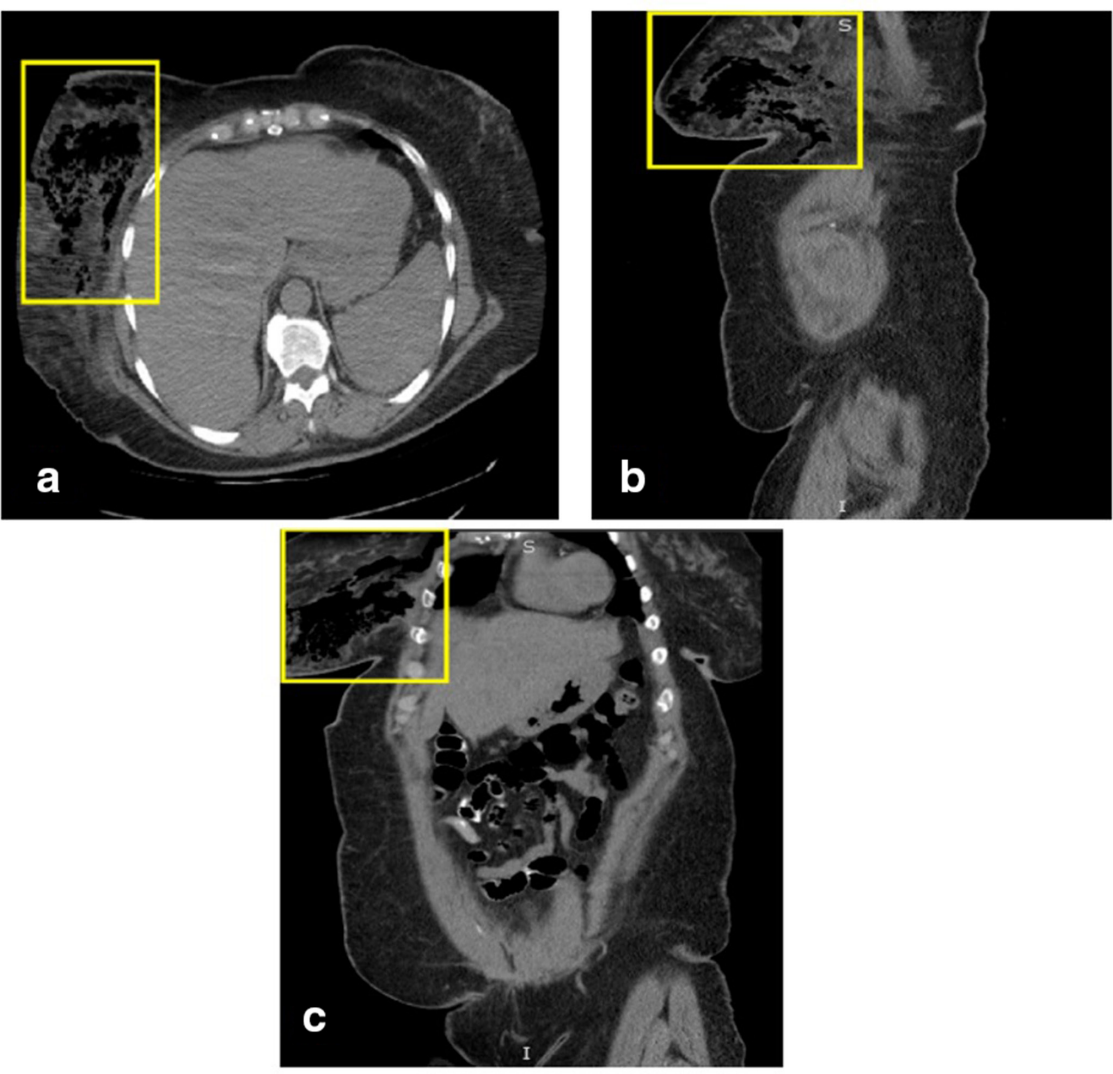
CT imaging displays the reported inflammatory changes and subcutaneous emphysema of the right breast. A displays an axial view with the yellow box demonstrating significant inflammatory changes and subcutaneous emphysema in the right breast. B displays a sagittal view with the yellow box highlighting subcutaneous emphysema in the right breast and inflammatory changes. Finally, C highlights the coronal view of the CT abdomen pelvis with the yellow box signifying the area of subcutaneous emphysema in the right breast and the abdominal wall.
